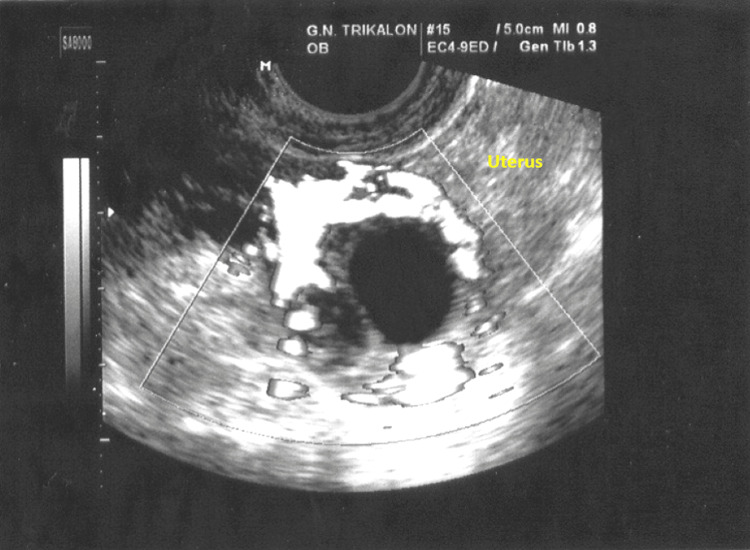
Transvaginal Doppler ultrasound imaging of the interstitial ectopic pregnancy Increased blood flow is an important criterion in the preoperative diagnosis of interstitial ectopic pregnancy.
